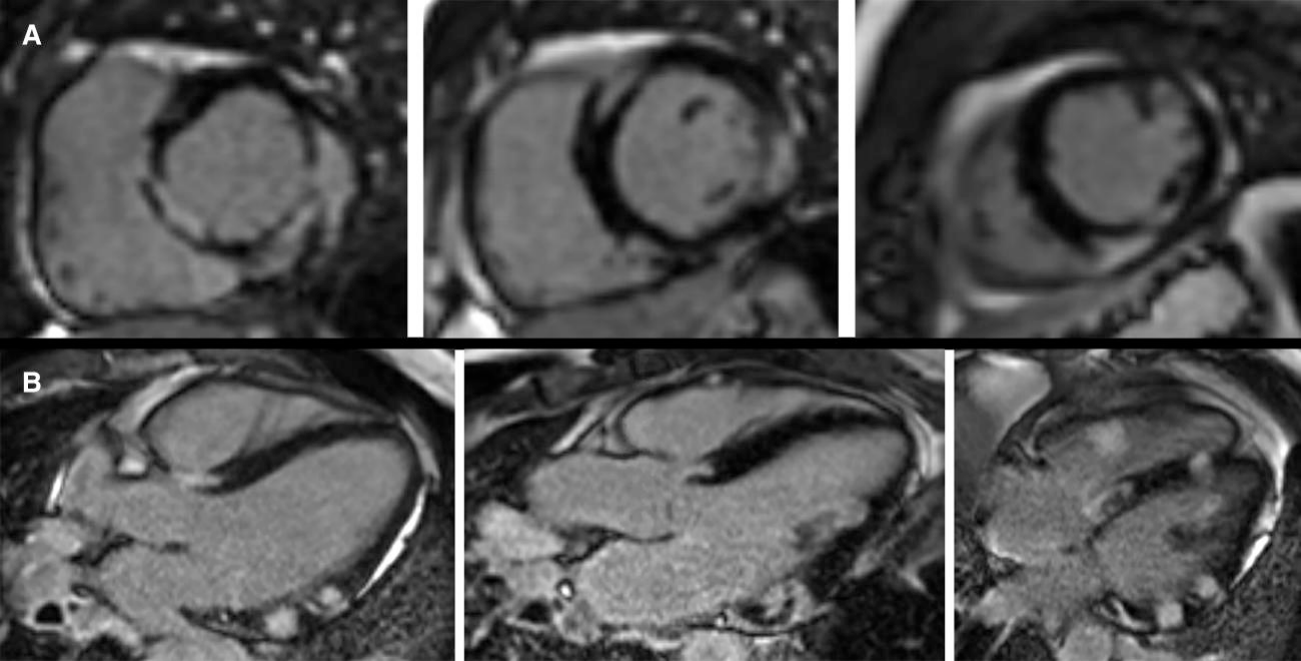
Cardiac magnetic resonance imaging short-axis (*A*) and long-axis views (*B*) showing extensive late gadolinium enhancement in a multifocal, non-coronary distribution involving subepicardial, subendocardial, and midwall layers.
